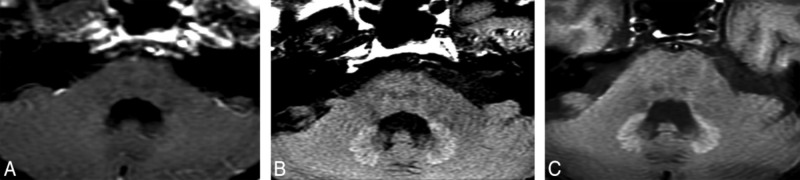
A patient with optical nerve pilocytic astrocytoma who treated with 5400-cGy radiation therapy and underwent 25 times CE-MRI scans show hyperintensity of the DN on unenhanced T1WI. DN initially appears as slightly hyperintense (A). During the follow-up studies performed at 5 (B) and 8 (C) years after the initial hyperintense appearance of the DN, the signal intensity of the DN become more obvious. Image courtesy of Dr. Mehmet Emin Adin, Russell H. Morgan Department of Radiology, Division of Neuroradiology, Johns Hopkins Medical Institutions, Baltimore, MD. Images and text reproduced from reference 1 with written permission from AJNR.
